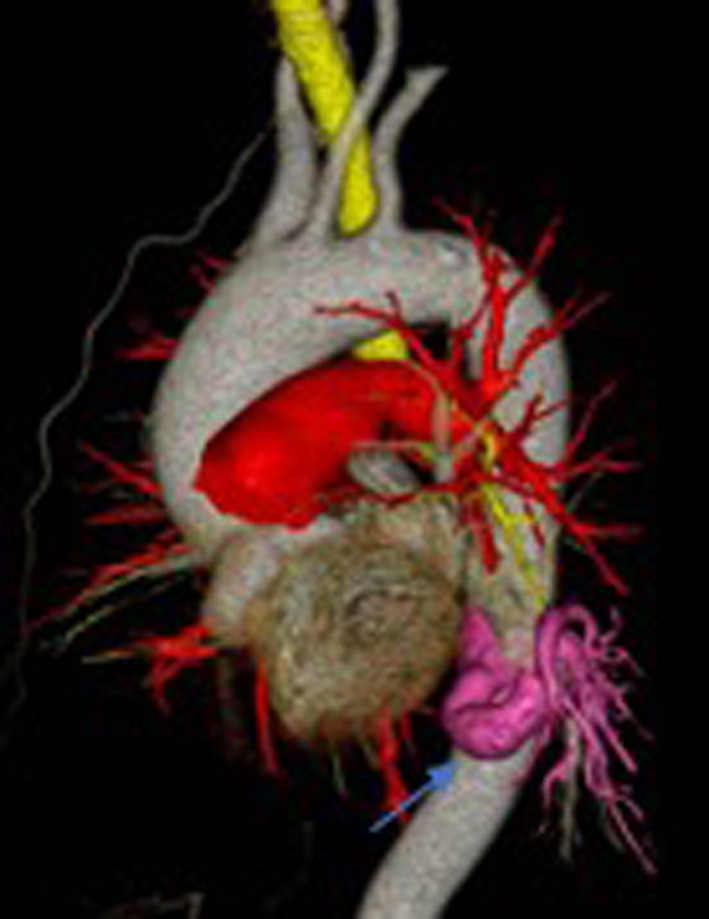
Three-dimensional CT image of the aberrant artery arising from the descending aorta(arrow)
